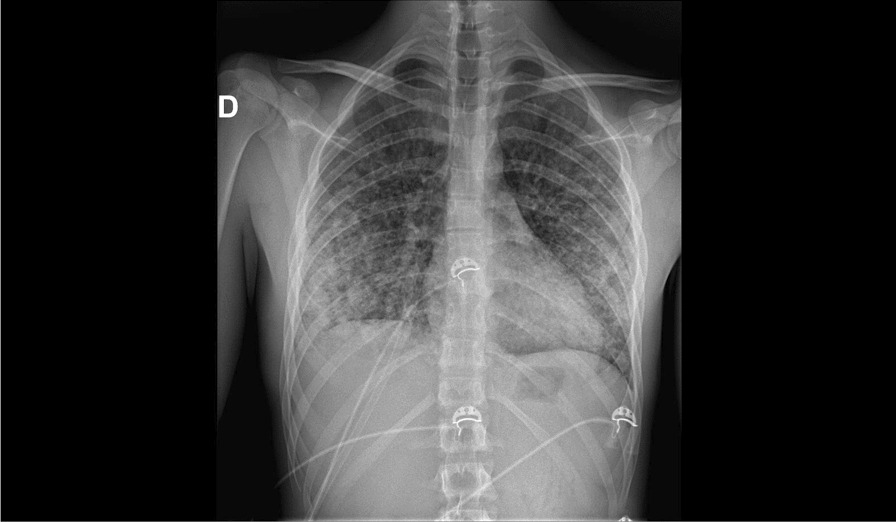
Chest radiography on arrival at the emergency room (H0): bilateral alveolar interstitial syndrome
